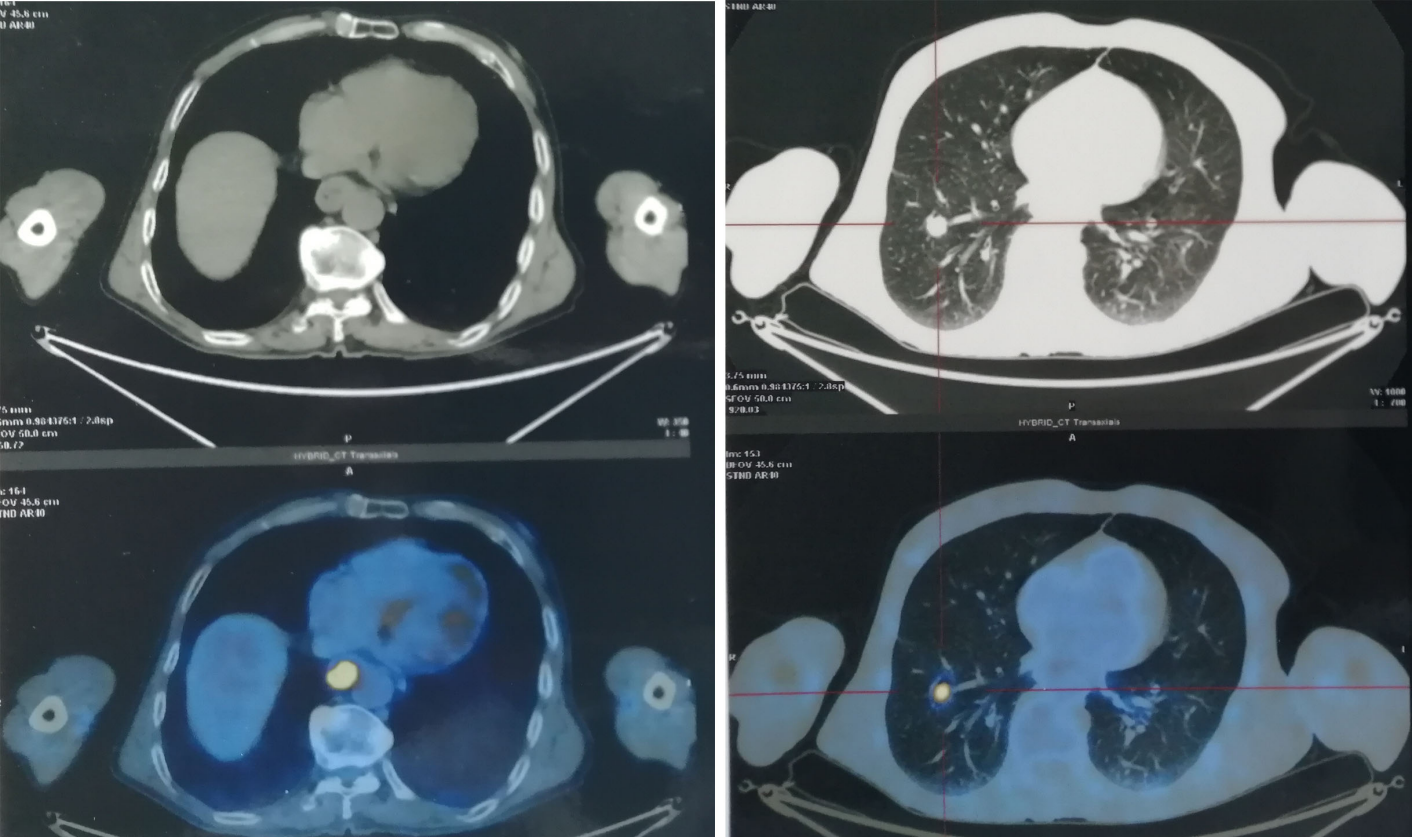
The PET/CT images showed a fludeoxyglucose avid primary mass of esophagus with pulmonary metastasis before treatment.
